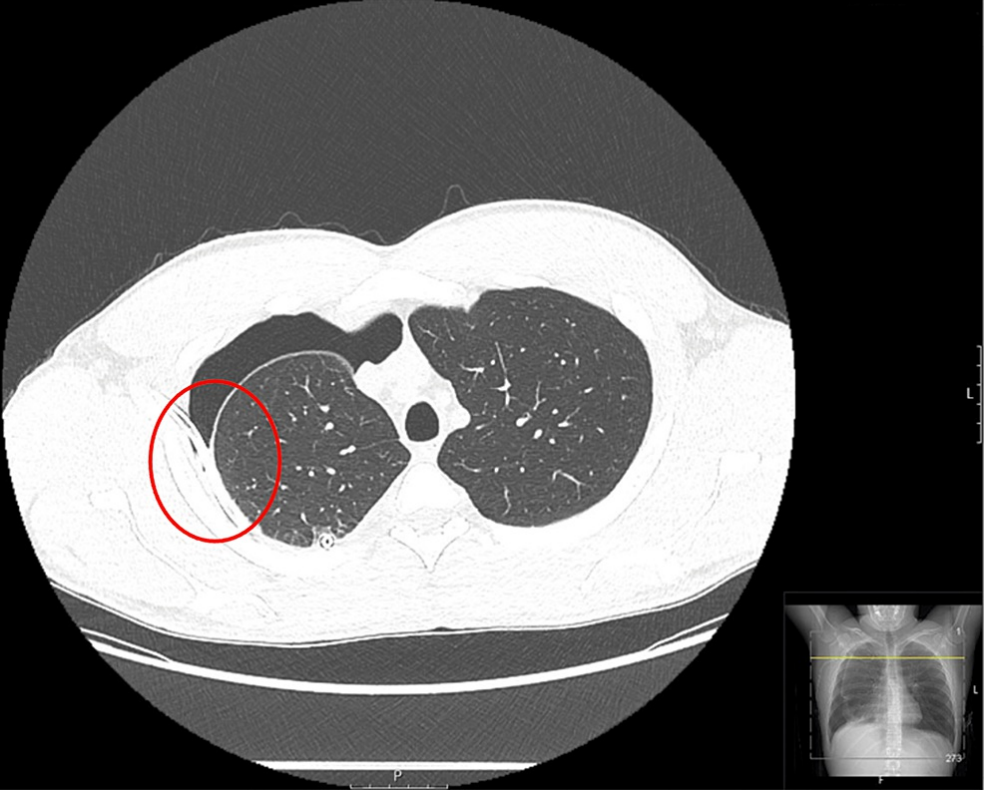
Chest CT without contrast of the upper lungs performed four days after initial CT Seen is a moderate to large, loculated hydropneumothorax that has improved. The right pleural effusion with locules of air is also indicated (red circle).
